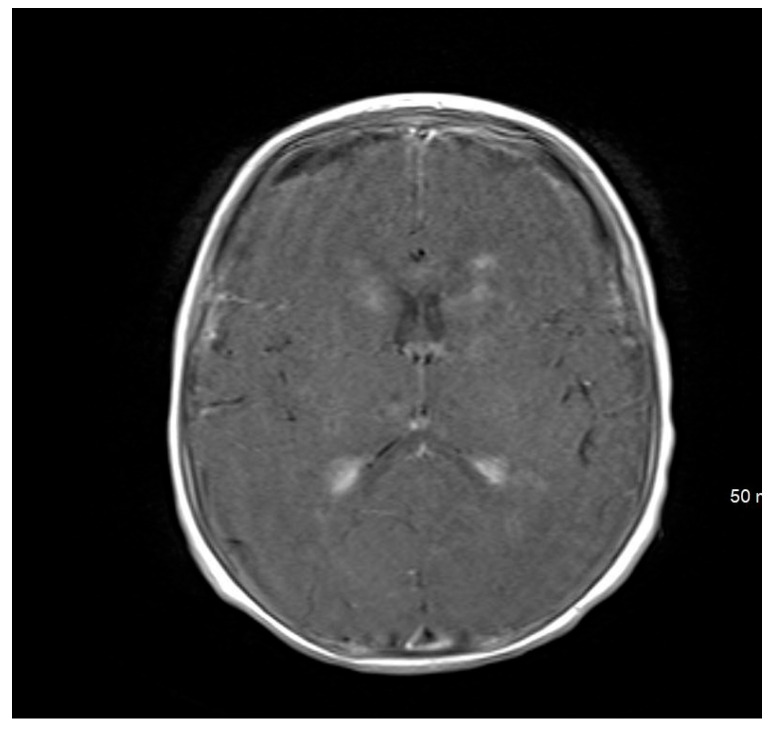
MRI brain scan showing meningeal enhancement.
